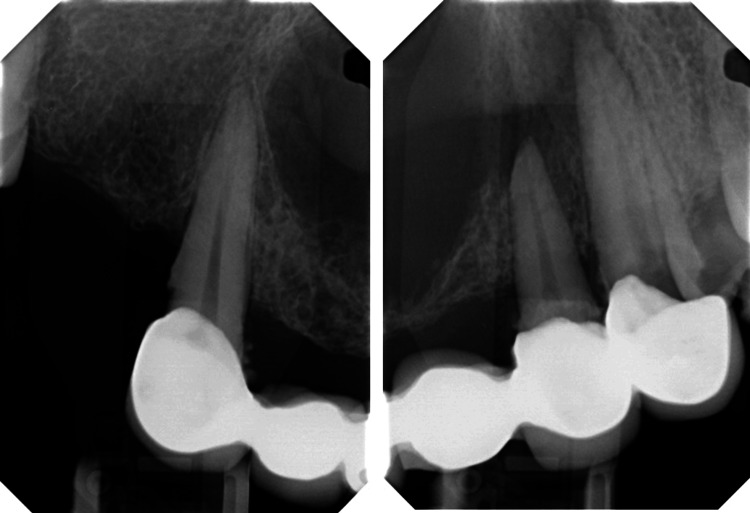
Periapical radiograph of the teeth associated with the cysts
